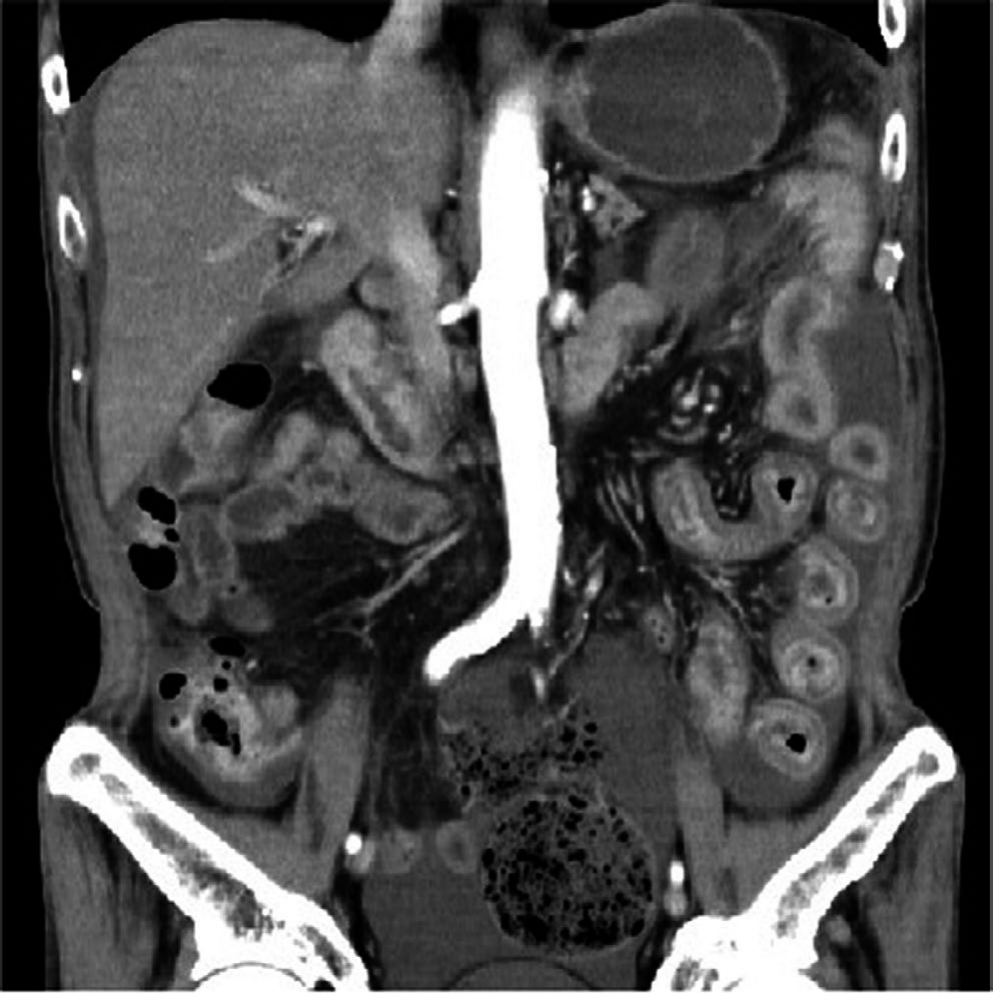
Abdominal computed tomography shows wall thickening of the duodenum and small intestine, and mild to moderate ascites
